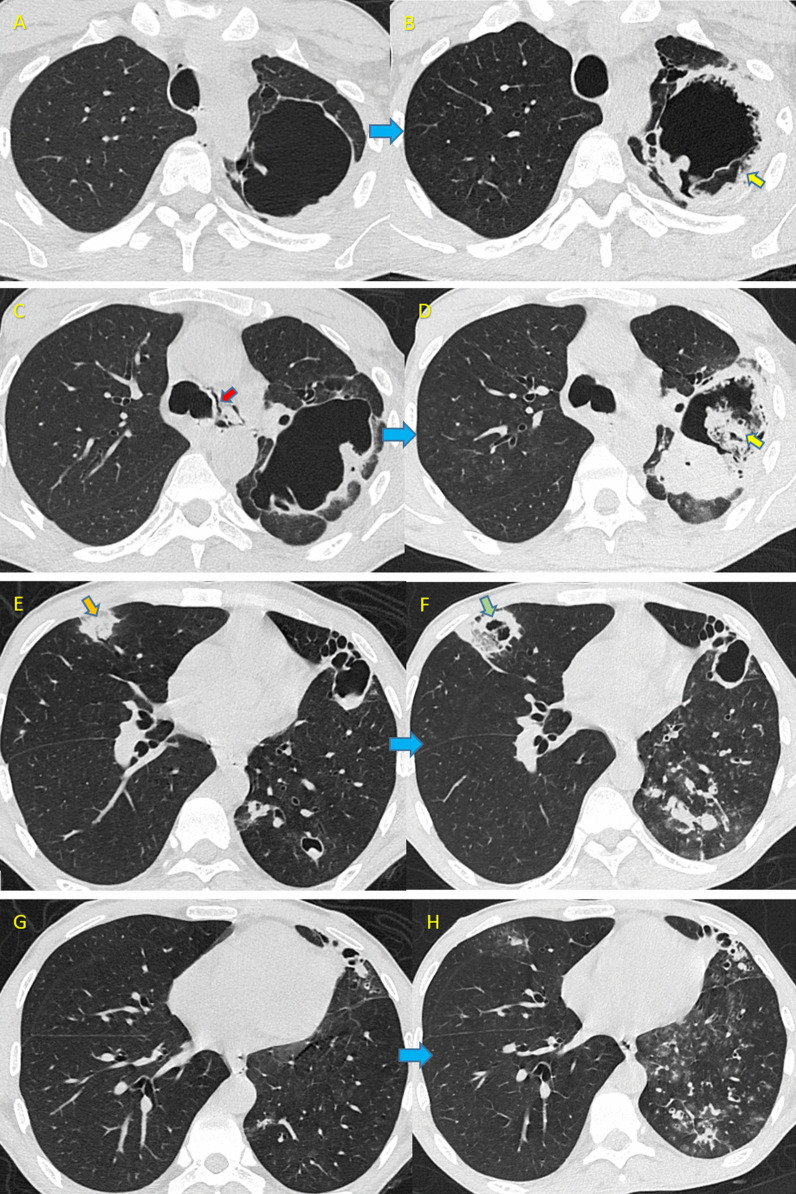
Axial HRCT scans showing imaging features of mucormycosis in case 2 of COVID-19. A–D CT axial images A and C show multiple cavitary lesions in the left upper and lower lobes. The repeat scans B and D show soft tissue attenuating growth (yellow arrows in B and D). Mild pneumomediastinum (red arrow in C) also noted. E, F A new cavity formed in the right upper lobe (green arrow in F) in pre-existing reverse halo sign (orange arrow in E). G, H Multiple soft tissue nodular opacities developed especially in left lower lobe in H in comparison with G
